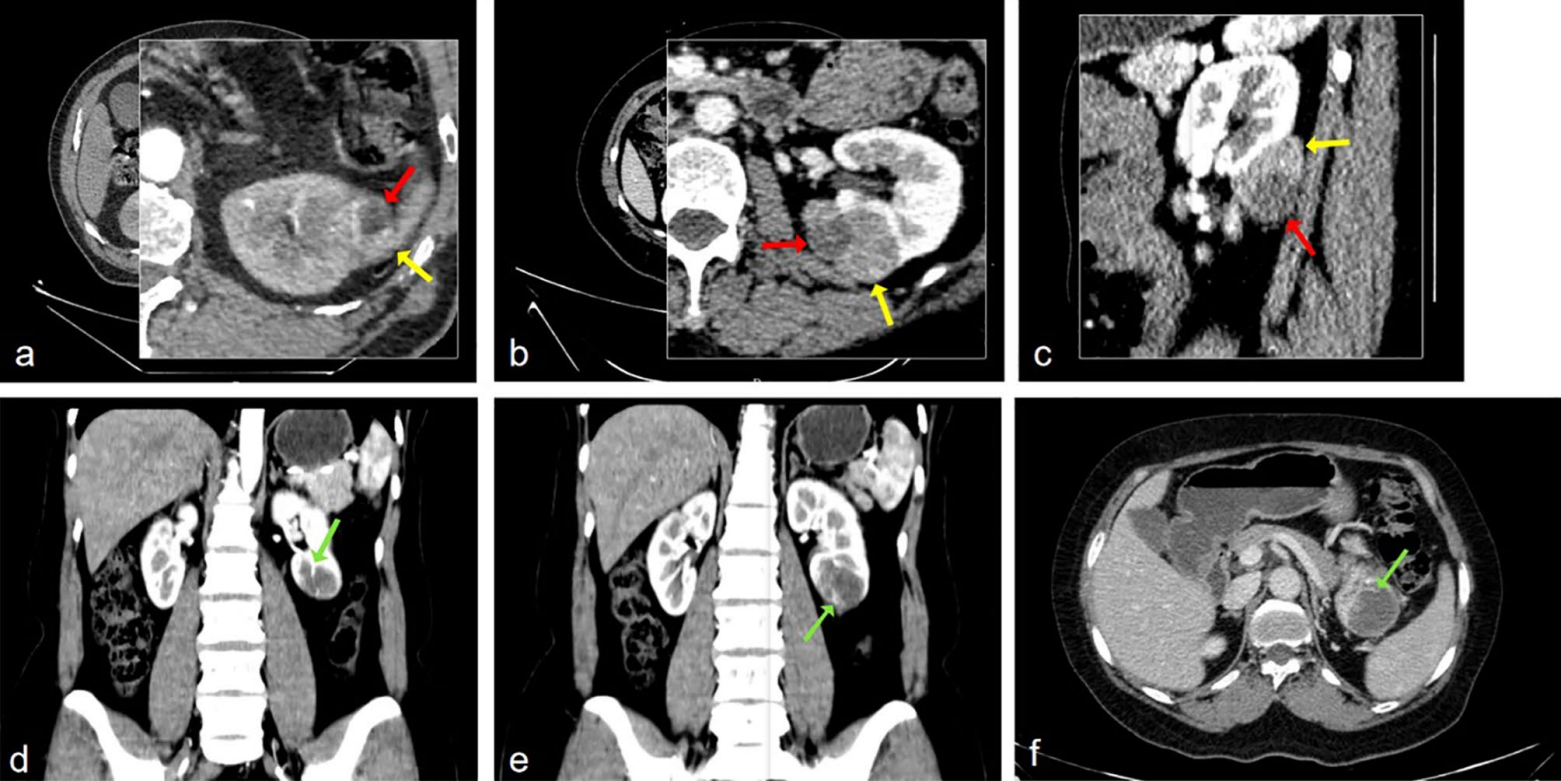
(a,b,c) Postcontrast CT: cystic (red arrow) and solid (yellow arrow) components of unilocular cystic clear cell RCC. (e,f,g) irregular internal structures (green arrow) in the unilocular cystic papillary RCC.
